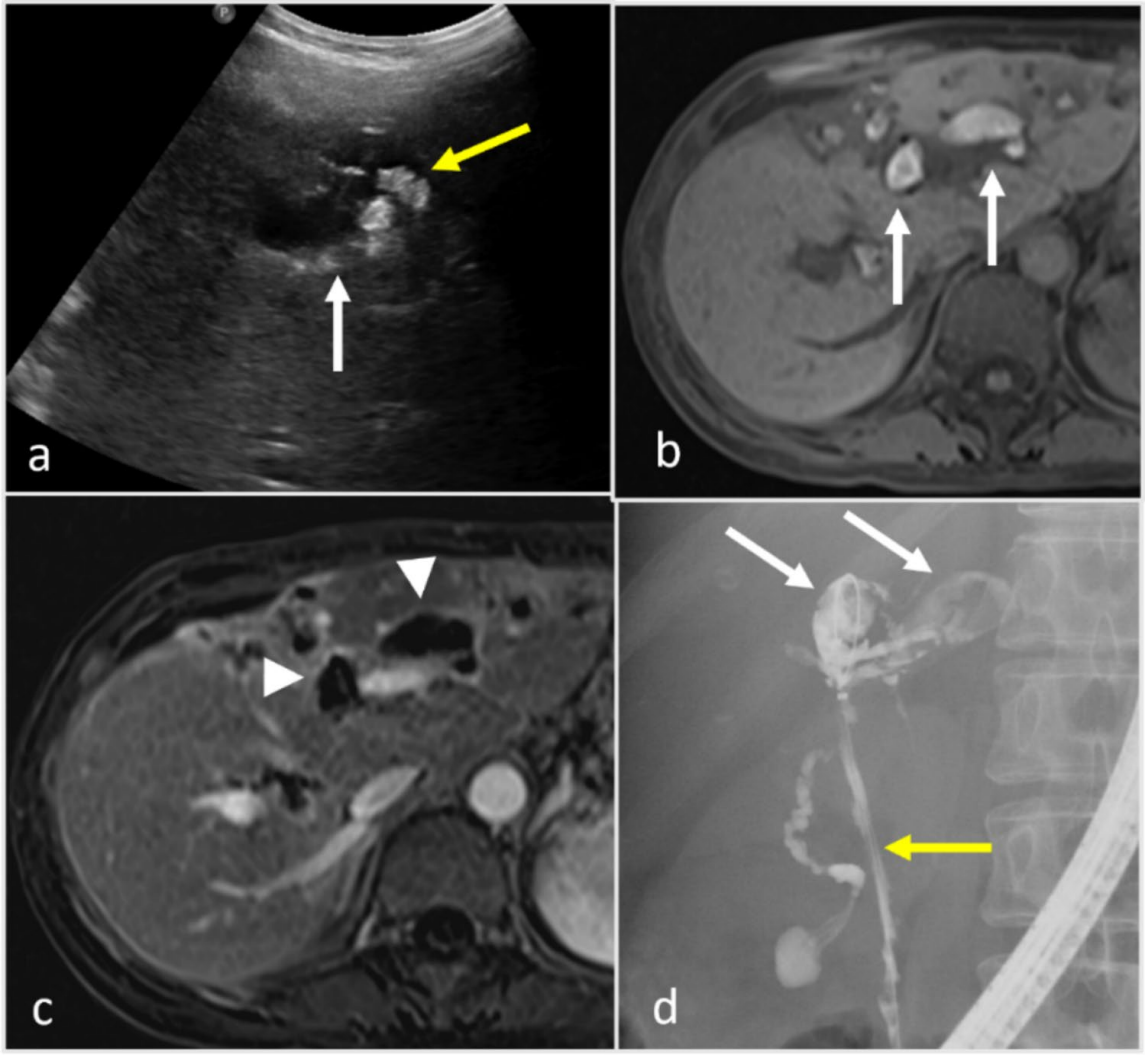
Intrahepatic bilomas in a 60-year-old female with history of Crohn's disease and primary sclerosing cholangitis presenting with elevated bilirubin. Ultrasound of the left hepatic lobe (a) shows a dilated intrahepatic duct (white arrow) containing debris (yellow arrow). Subsequent MRI and MRCP (MRCP not shown) showed multiple intrahepatic ducts with dilatation and stricturing. On pre T1-weighted MR imaging (b) dilated ducts in the left hepatic lobe are seen containing intrinsic T1 hyperintense debris (white arrows). Subtraction post-contrast MR image (c) demonstrates peri-biliary enhancement (white arrowheads) consistent with active cholangitis. The patient underwent ERCP (d) which confirmed intrahepatic bilomas with debris (white arrows). Long segment stricturing of the extrahepatic duct can also be visualized (yellow arrow)
